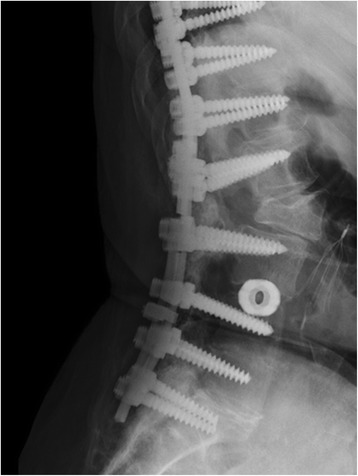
Lateral X-rays demonstrating fracture of rod at osteotomy site
